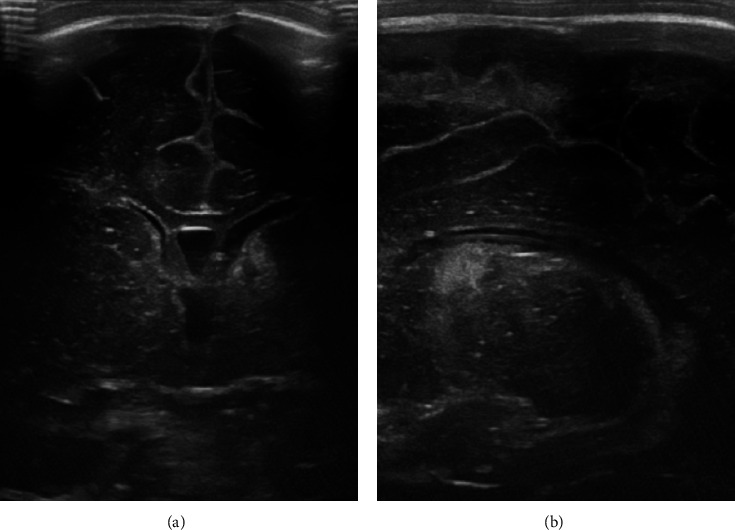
Cerebral ultrasound imaging. Ultrasound imaging shows demarcation of the increased echogenicity in the left subependymal area and basal ganglia: (a) coronal plane and (b) parasagittal plane.
